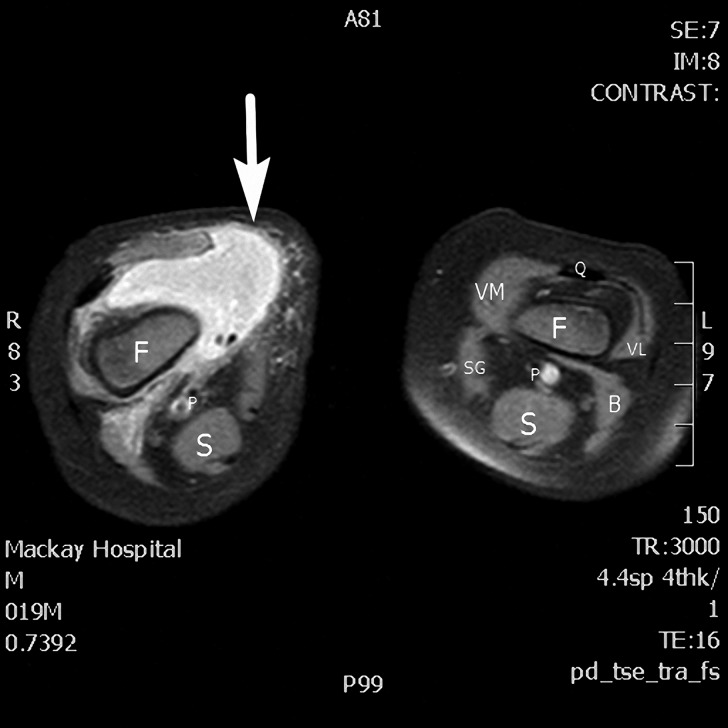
The axial view of T2-weighted knee magnetic resonance imaging shows abscess formation in the right suprapatellar bursa (arrow). The anatomic structures are labeled—B = biceps femoris, F = femur, S = semitendinosus and semimembranosus muscles, P = popliteal artery and vein, Q = quadriceps tendon, SG = sartorius and gracilis muscles, VL = vastus lateralis, VM = vastus medialis.
